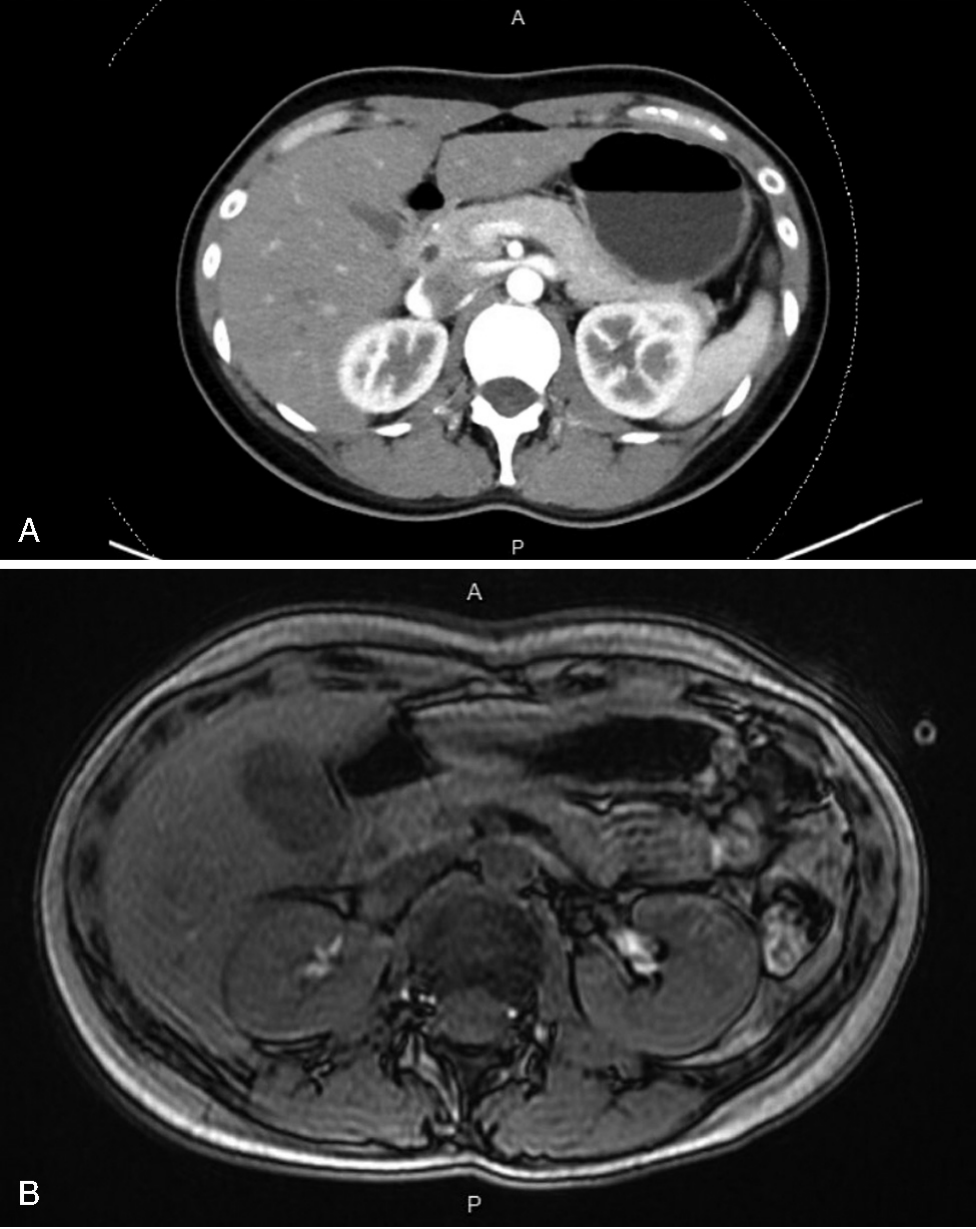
(A) Imaging result of abdominal computed tomography revealed no evidence of no evidence of pancreatic tumor nor insulinoma. (B) Imaging result of abdominal magnetic resonance imaging revealed no evidence of pancreatic lesion or insulinoma.
